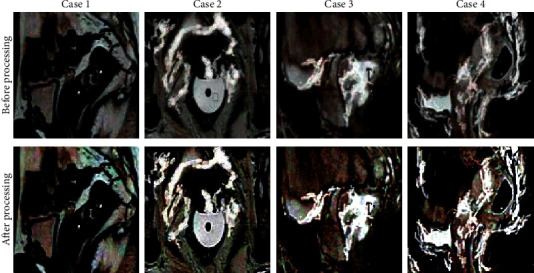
MRI images of typical cases.
